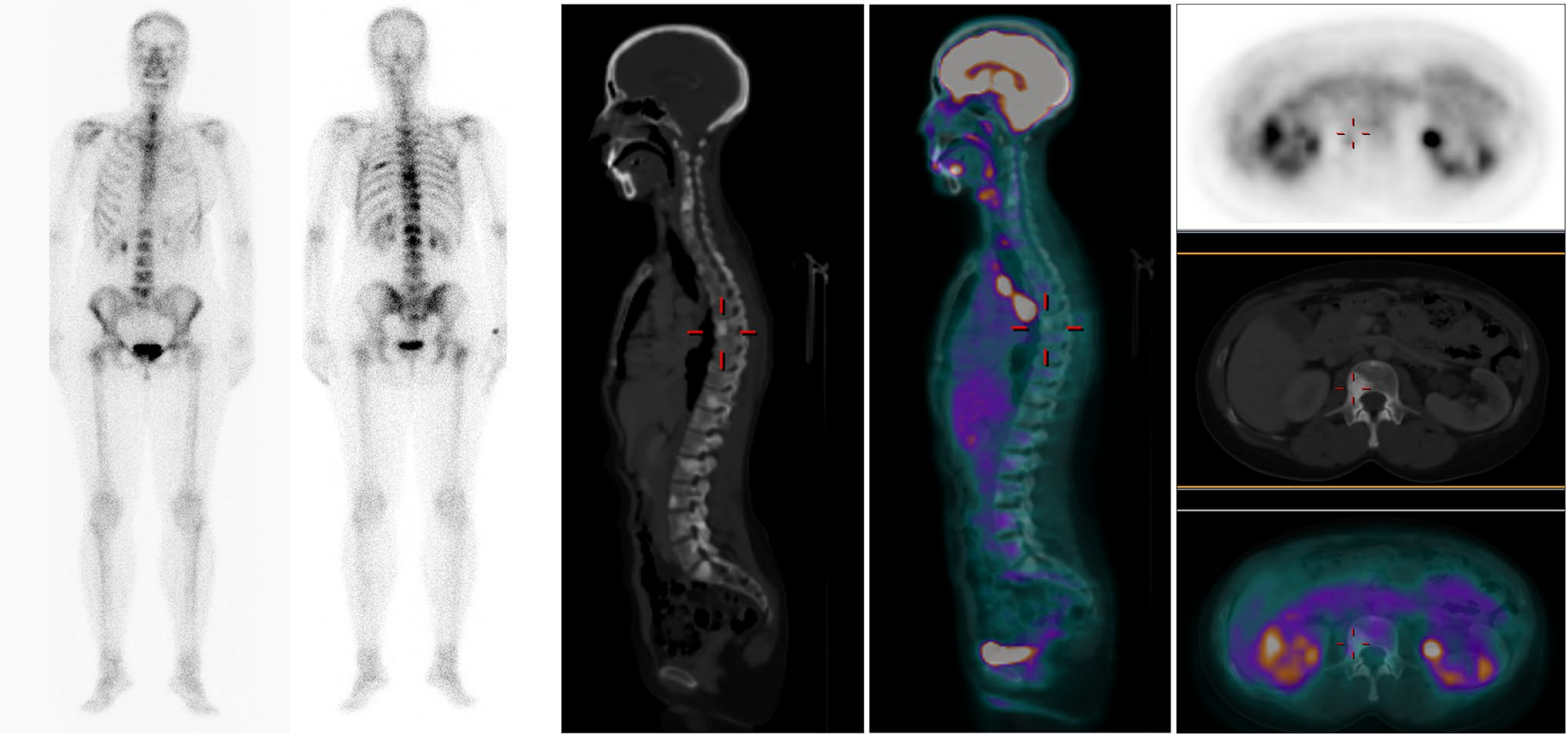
Added value of PET/CT in the characterization of treated secondary bone lesions. A 53-year-old woman, diagnosed with infiltrating ductal breast carcinoma, treated with surgery, chemotherapy, and radiotherapy. Bone scintigraphy and CT showed osteoblastic lesions suspicious for metastases that did not respond to treatment. In this scenario, FDG-PET/CT image makes it possible to rule out signs of tumor viability in skeletal lesions, classifying them as old lesions with a complete response to treatment
